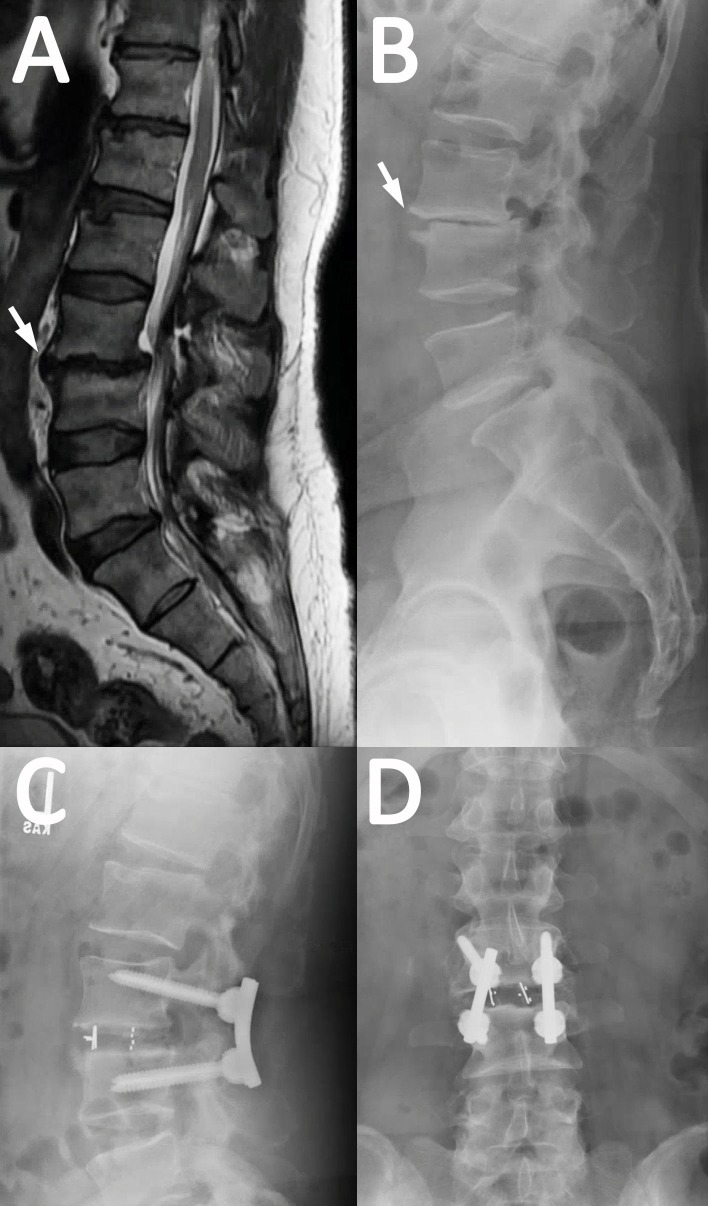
Representative case example using CBT A 58-year-old male presents with acute progression of chronic back pain with radiculopathy and subjective lower extremity weakness. He underwent an L3 laminectomy, L3/4 transforaminal lumbar interbody fusion (TLIF), and posterior spinal fusion. (A) Preoperative magnetic resonance imaging and (B) x-rays show spondylolisthesis of L3 on 4 and spinal stenosis (arrow). (C) Lateral and (D) anteroposterior x-rays after L3/4 TLIF using the cortical bone technique (CBT) are shown.
